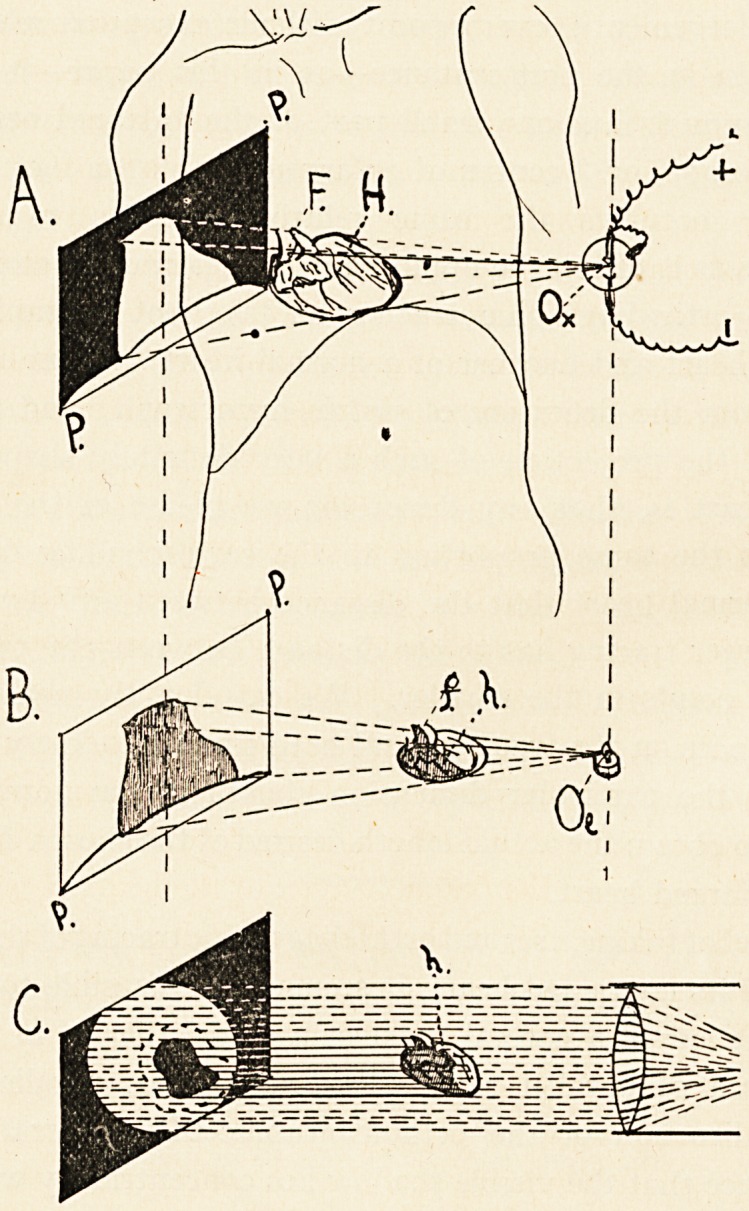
# Radiographic Estimation of Simple Enlargement by Means of Visible Shadows
